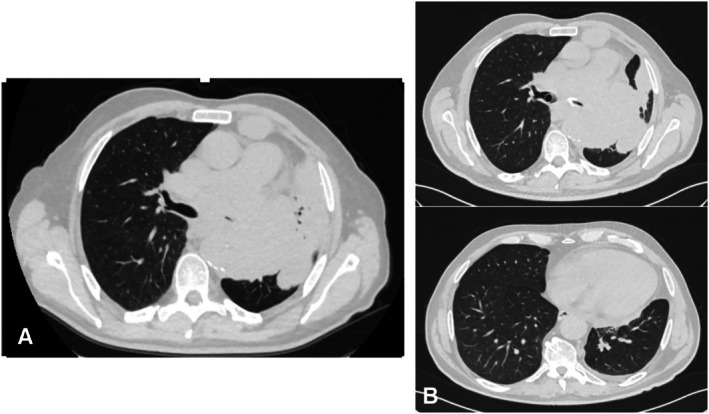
Multislice spiral computed tomography (MSCT) scan of the chest were performed to provide reliable data for stent selection and size customization. (A) Pre stent‐placement and (B) post stent placement.
